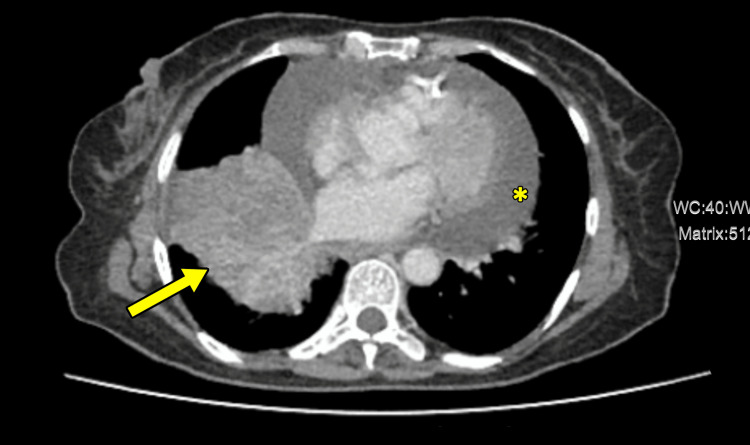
CT chest shows a right lower lobe mass lesion obstructing the lower lobe bronchus, causing collapse of the right lower lobe, suggestive of lung malignancy (arrow). Additionally, there is a significant pericardial effusion (asterisk). CT, computed tomography
